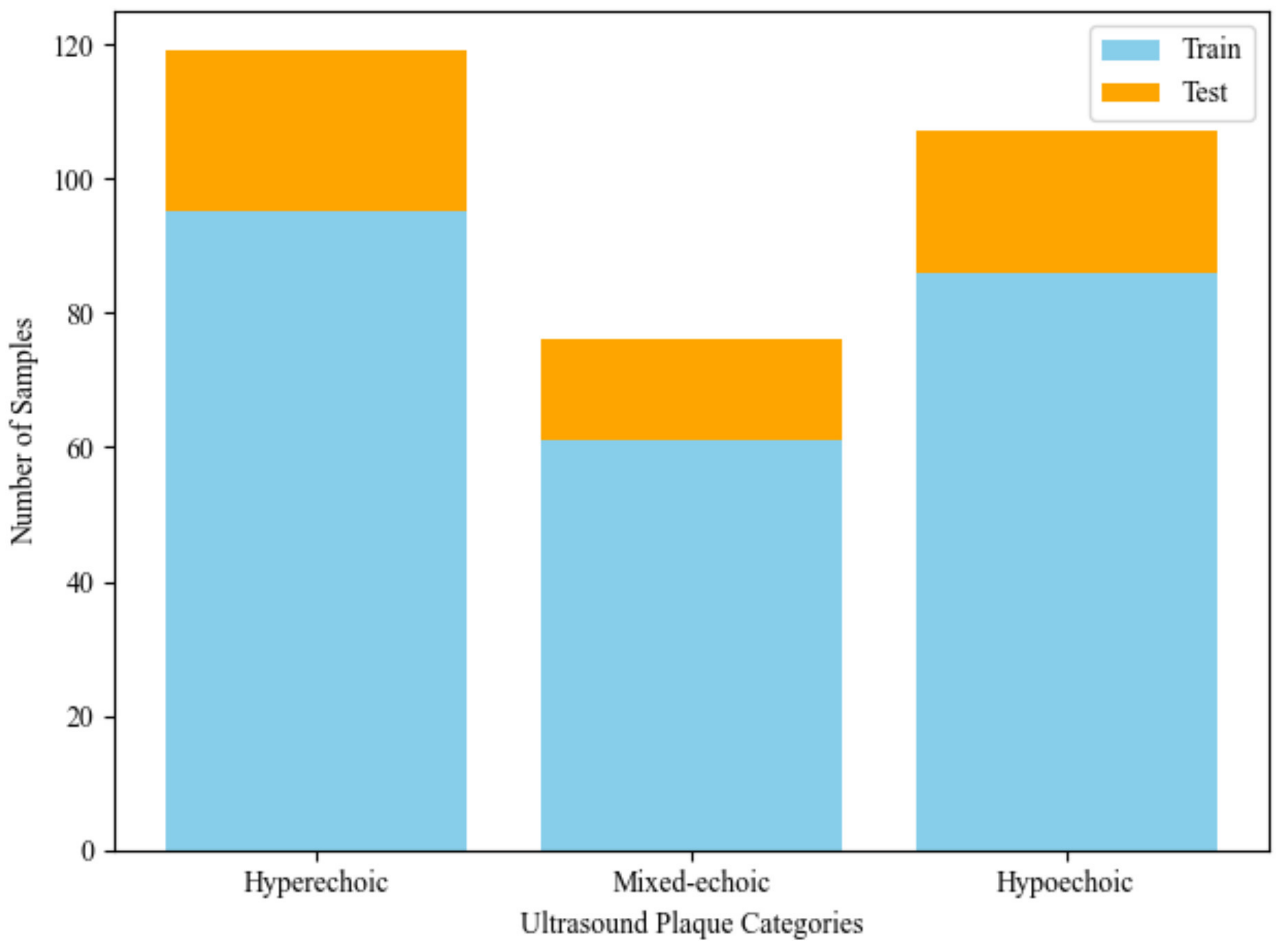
Distribution of plaque types across training and test sets in the ultrasound video dataset.
